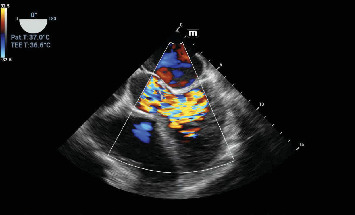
Transesophageal echocardiography confirmed a significant aortic regurgitation, with no indications of aortic root abscess or aneurysmal rupture.
